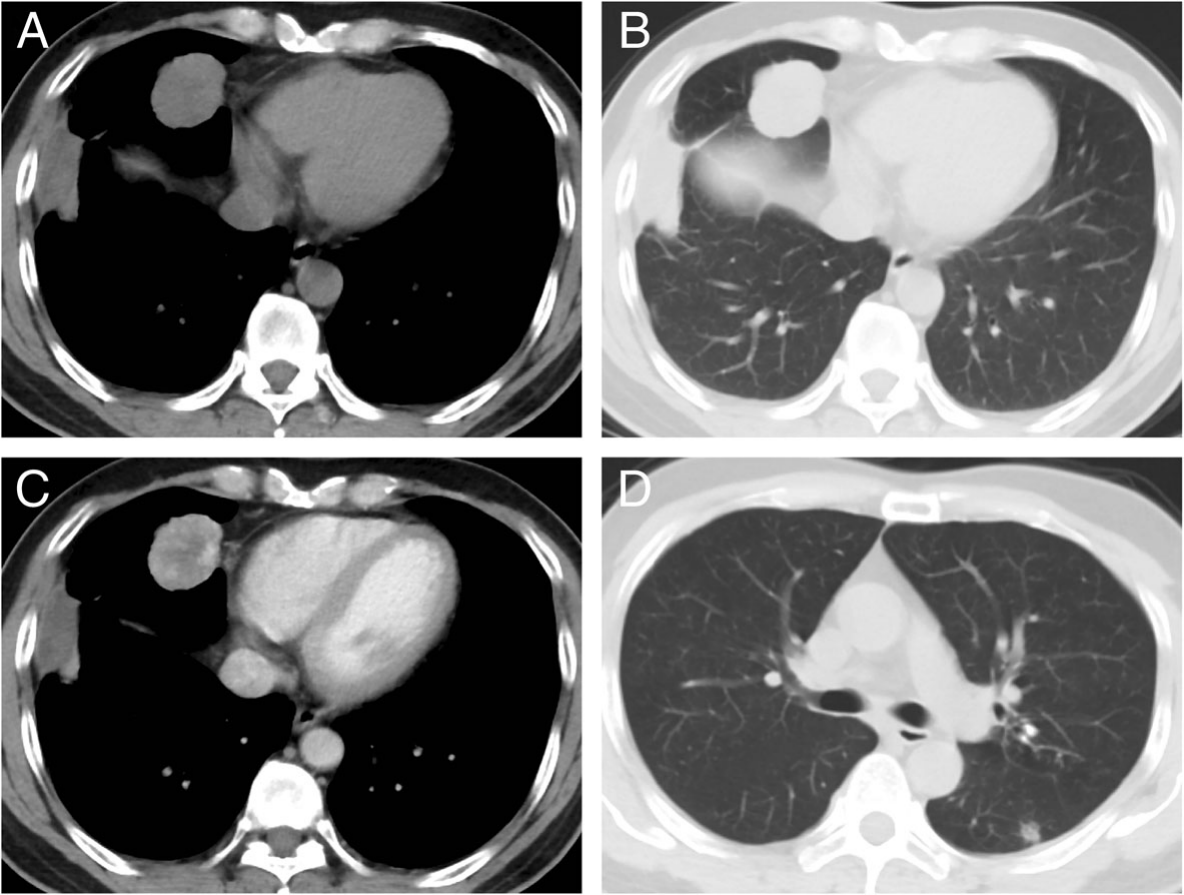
Computed tomography (CT) scan of the lung tumor. a, b A larger lesion was located in the right middle lobe with smooth boundary measured 4 cm × 3.5 cm × 2 cm, accompanied by heterogeneous enhancement (c). d Another small nodule about 11 mm was located in the left lower lobe with lobulated shape and spicules of margin
